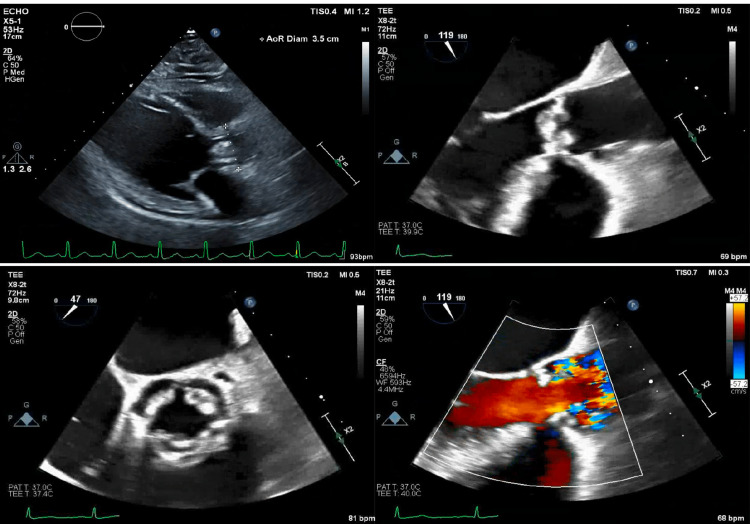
Thickened aortic valve cusps as seen on transthoracic echocardiogram (top left) and transesophageal echocardiogram (top right) Transesophageal echocardiogram displaying aortic valve echo-densities on the parasternal short access view (bottom left) and aortic regurgitation (bottom right).
